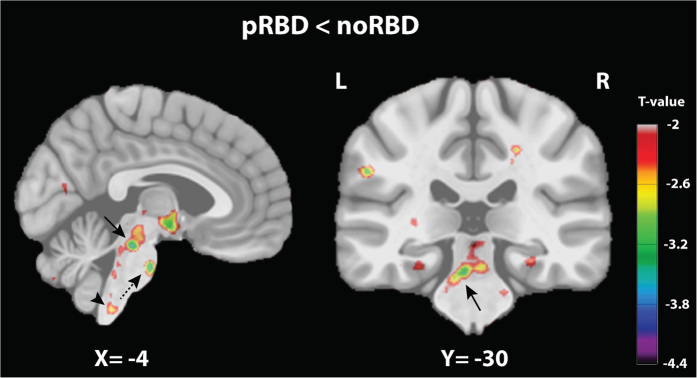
Volume change comparison between PD patients with (pRBD) and without (noRBD) probable RBD in the brainstem. Thresholded *t* maps (at *P* < 0.05 corrected for multiple comparisons) superimposed onto Montreal Neurological Institute 152 brain imaging template (i.e., a normalized average of 152 3D T1-weighted MRI scans from a normative adult population). The color-coded bar represents t values and the display is thresholded at *P* < 0.05. Left, sagittal plane showing smaller volume in the pontomesencephalic tegmentum (PMT) (arrow), the ventral medulla (arrow head), and base of pons (dashed arrow). Right, coronal plane showing the bilateral smaller volume in the PMT area (arrow).
